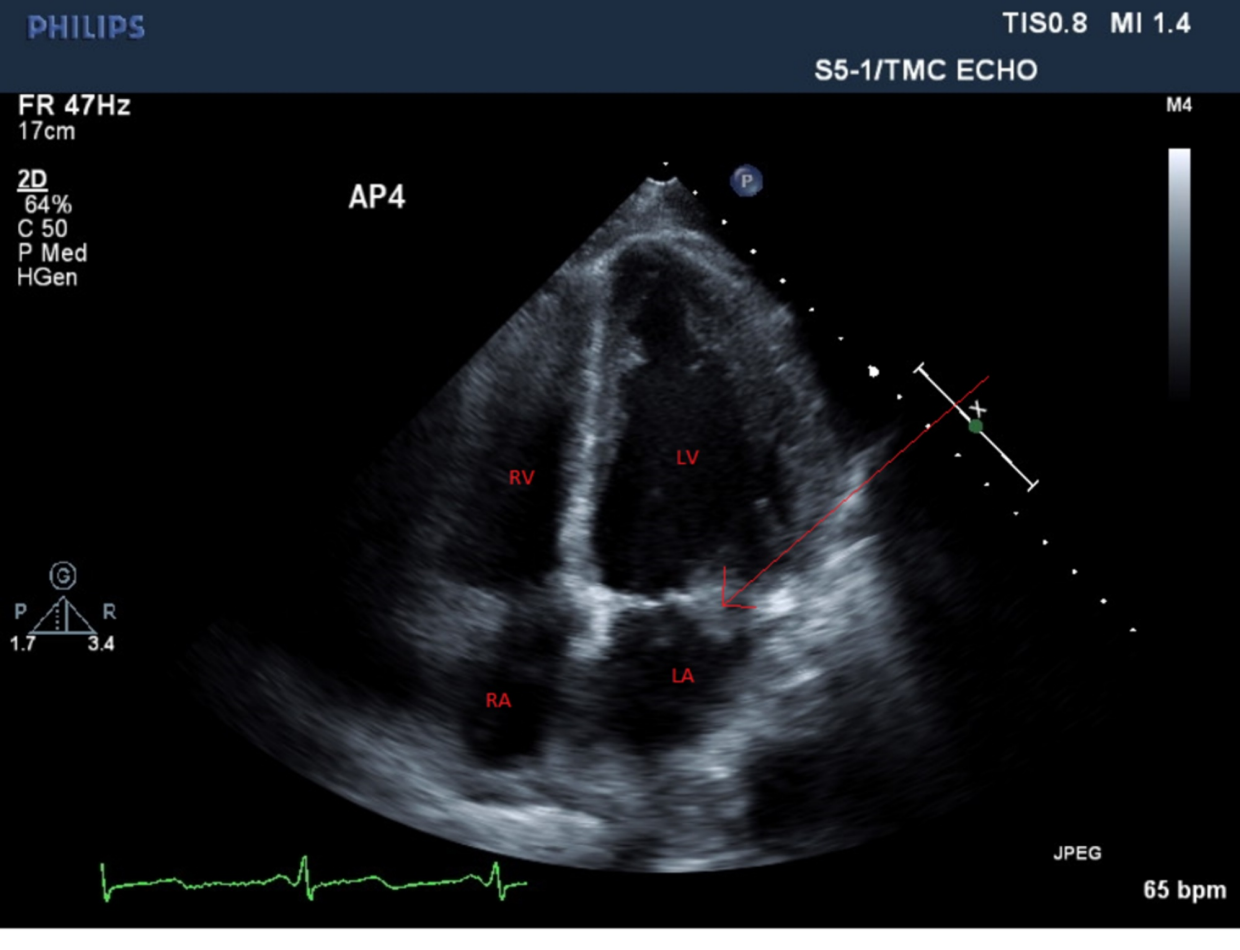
A four-chamber echocardiogram image showing a large mobile vegetation (arrow) on the mitral valve. RA: Right Atrium. RV: Right Ventricle. LA: Left Atrium. LV: Left Ventricle.
